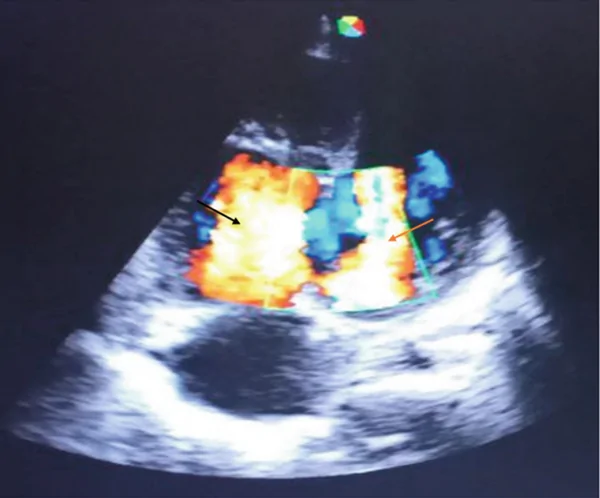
Two‐dimensional echocardiography showing membranous VSD with moderate to severe mitral regurgitation, mild mitral valve prolapse, severe tricuspid regurgitation, and severe pulmonary hypertension.
